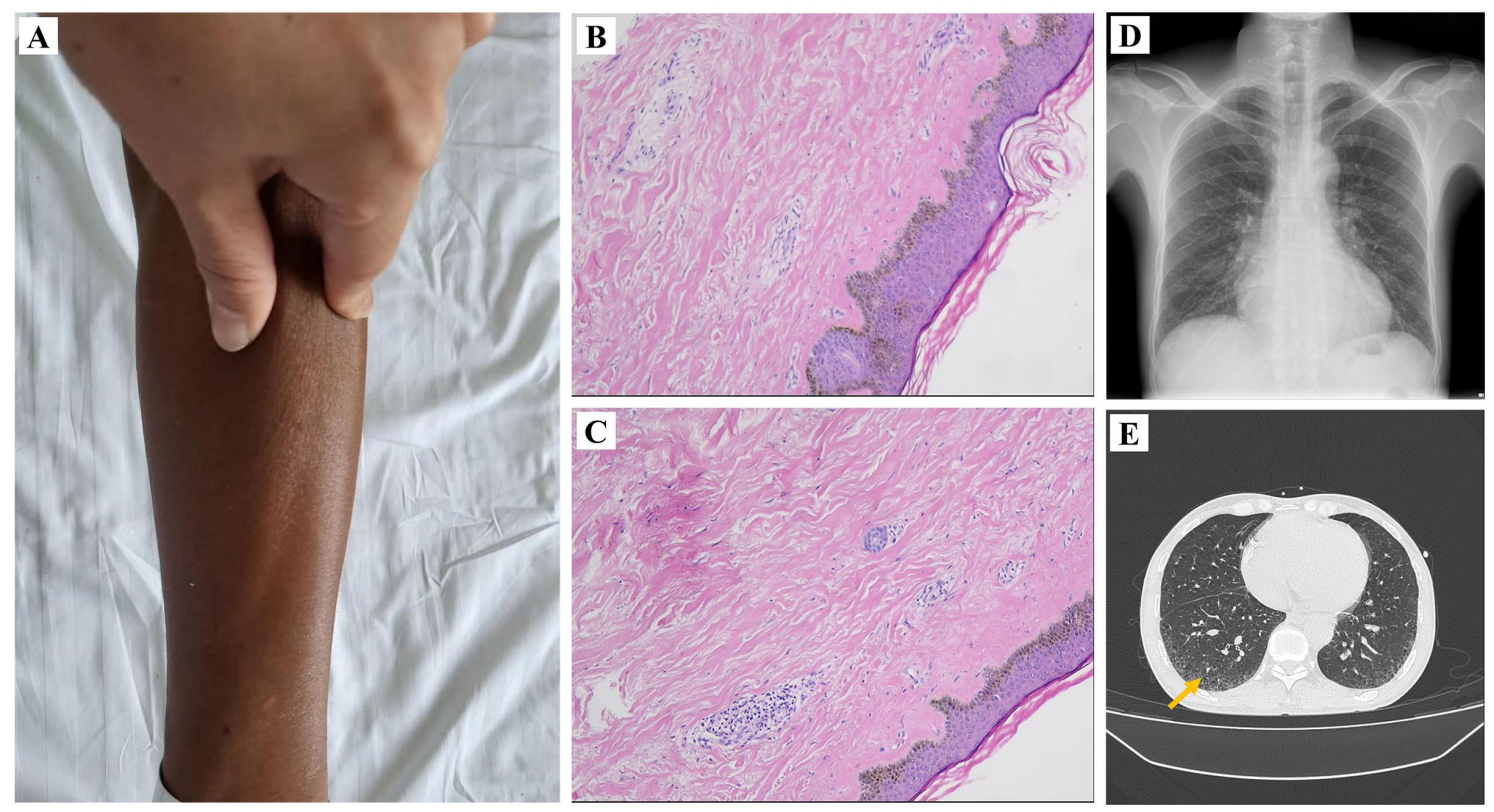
Features of skin appearance and pathology as well as lung imaging findings. The skin on the patient’s forearm presented as tight and dull (A). The pathological findings indicated dermal and subcutaneous thickening with fibrous tissue hyperplasia and focal chronic inflammation (B, C). Chest X-ray revealed thickened lung texture and slight enlargement of the right heart (D). Chest computed tomography (CT) showed pulmonary fibrosis in the right lower lung (superior segment) (E).
